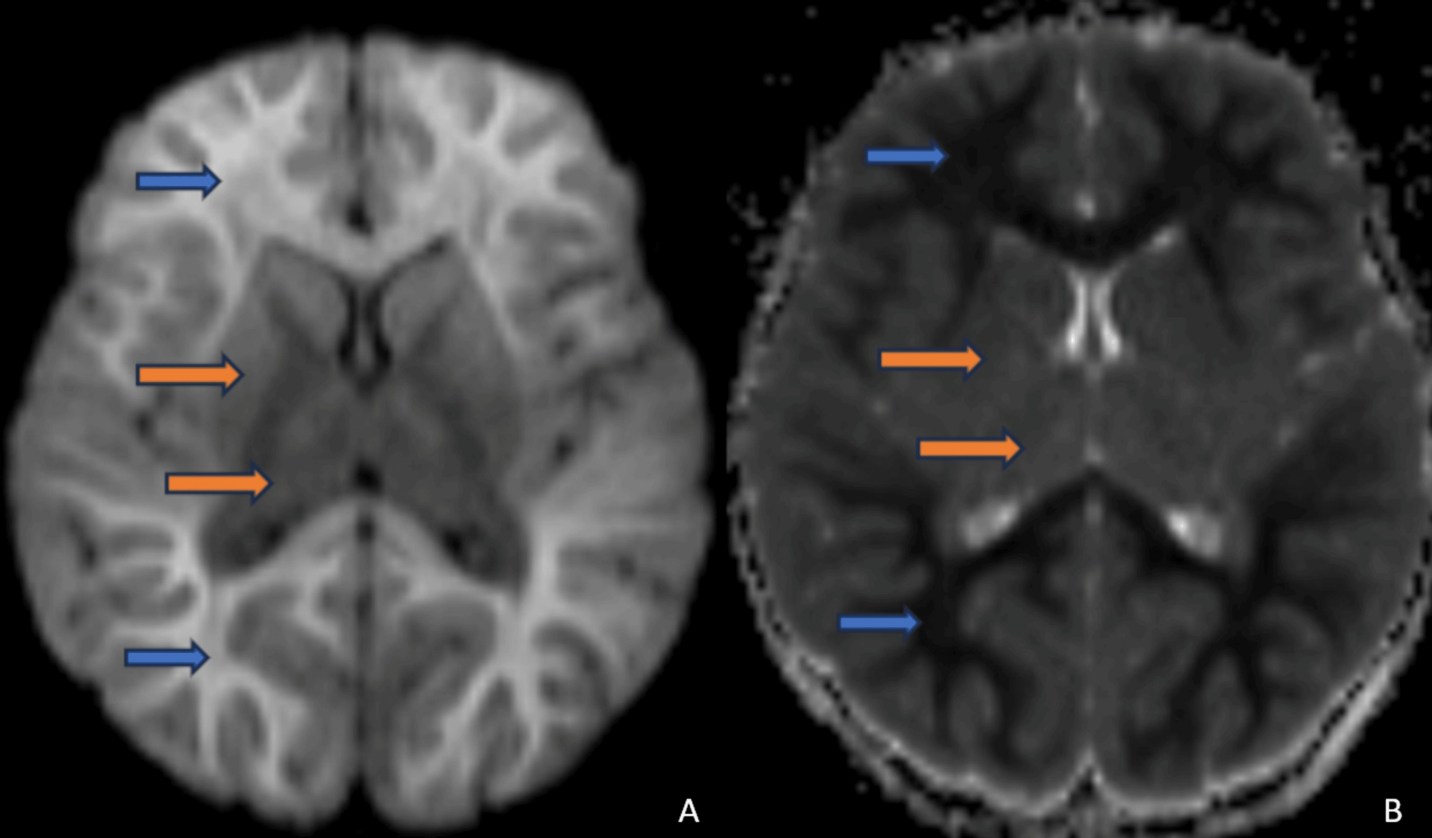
Diffusion weighted images and corresponding ADC images show marked diffusion restriction in form of high signal on DWI [1A] with corresponding low ADC value [1B] in subcortical white of bilateral frontal and parietal lobes (Blue arrow). The basal ganglia and thalamus are spared (orange arrow). ADC: Apparent diffusion coefficient, DWI: Diffusion weighted imaging
